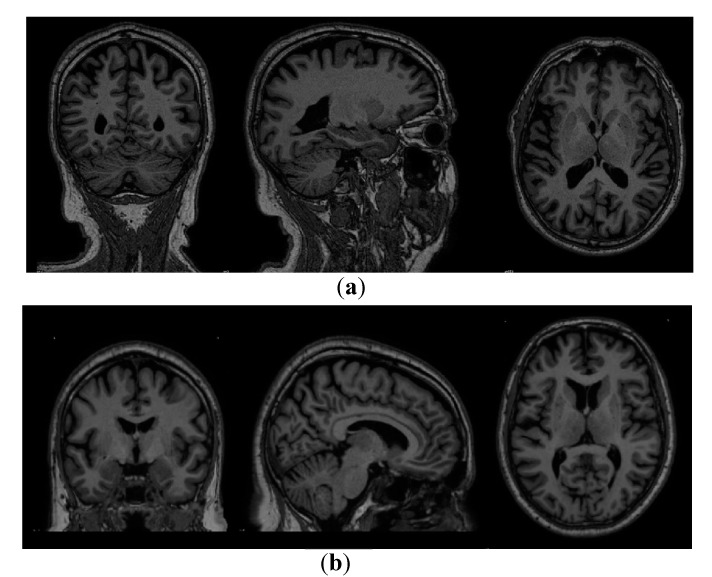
Sample scan pre and post-registration. (a) MRI scan before registration (b) MRI scan after registration.
